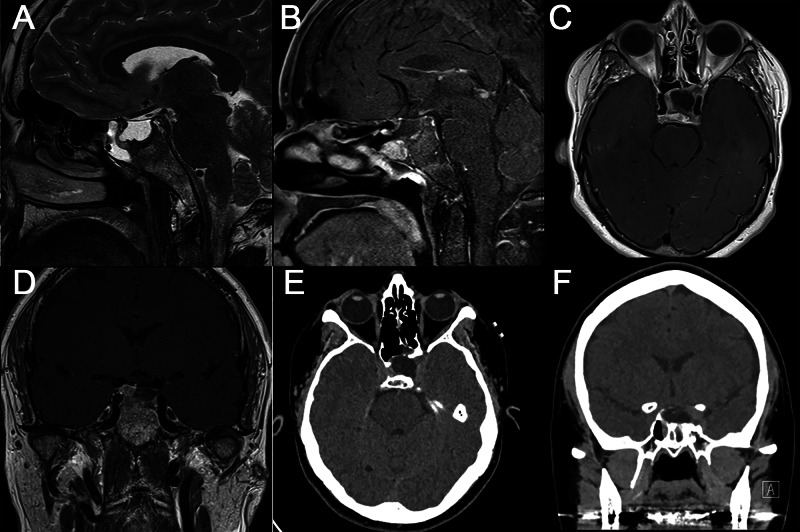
Preoperative sagittal T2-weighted (A), sagittal (B), axial (C), and coronal (D) contrasted T1-weighted MRI as well as axial (E) and coronal (F) CT scans showing CSF-filled sella, tumor in the sphenoid sinus, deviation of the pituitary stalk and gland, eroded anterior sella wall, and osseous destruction at the base of the sphenoid extending to the medial margin of the left cavernous sinus. MRI, magnetic resonance imaging; CT, computed tomography; CSF, cerebrospinal fluid
